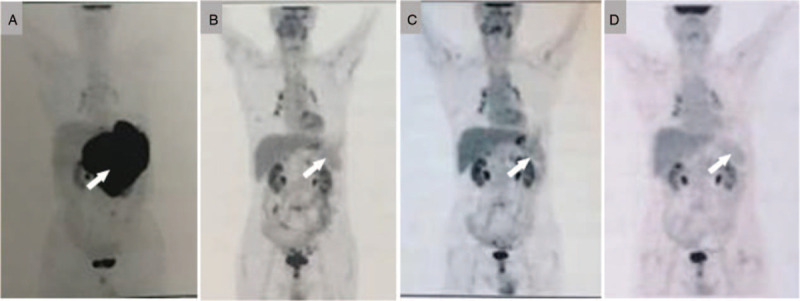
PET/CT scans of Case 2 during treatment: A, prior to initial treatment; B, partial response after 4 cycles of first-line chemotherapy; C, disease progression after 3 months of lenalidomide maintenance therapy; D, CR after 5 months of lenalidomide plus chidamide therapy. CT = computed tomography; CR = complete response; PET = positron emission tomograph.
